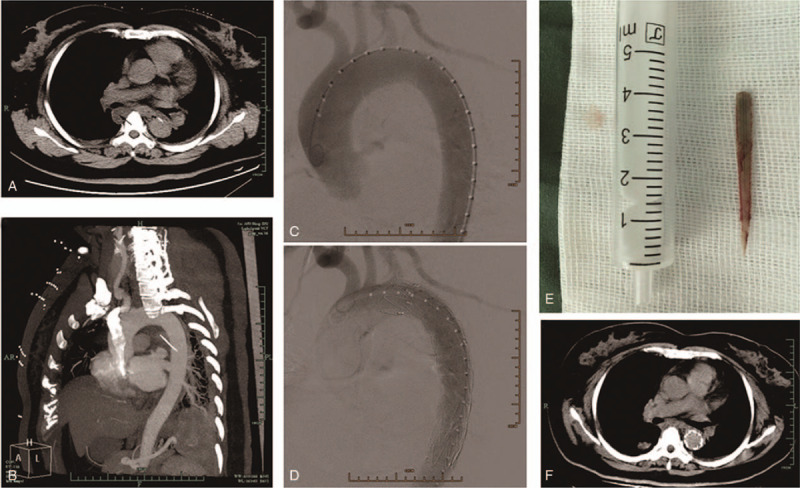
Representative images of case 2. (a) Chest CT at admission revealed a foreign body perforating the thoracic esophagus and aorta. Besides, an inferior right mediastinal cyst (arrow) was noted. (b) A subsequent CTA demonstrated a linear hyperdensity foreign body puncturing into the descending aorta, with no sigh of leakage of contrast agents and formation of hematoma. (c) The angiographic catheter was guided into the thoracic aorta and arteriography revealed no sign of pseudoaneurysm and leakage of contrast agent. (d) An endograft (28 × 150 mm, Medtronic, Inc., USA) was placed inside the descending aorta. (e) The removed fishbone during the surgery. Then, mediastinal debridement and copious irrigation was performed. (f) Chest CT scan at 1 month follow-up confirmed successful closure of the fistula.
